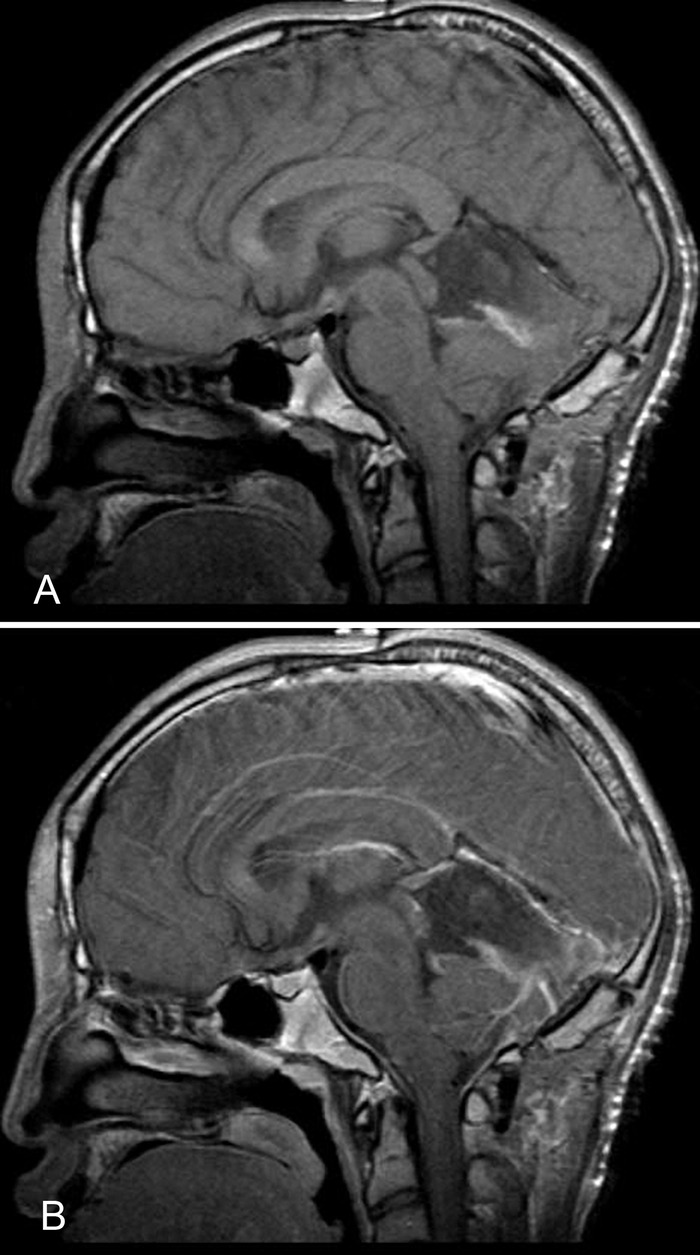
Magnetic resonance imaging without contrast (A) and with contrast (B) after surgical treatment showed postoperative changes and no evidence of residual tumor.
